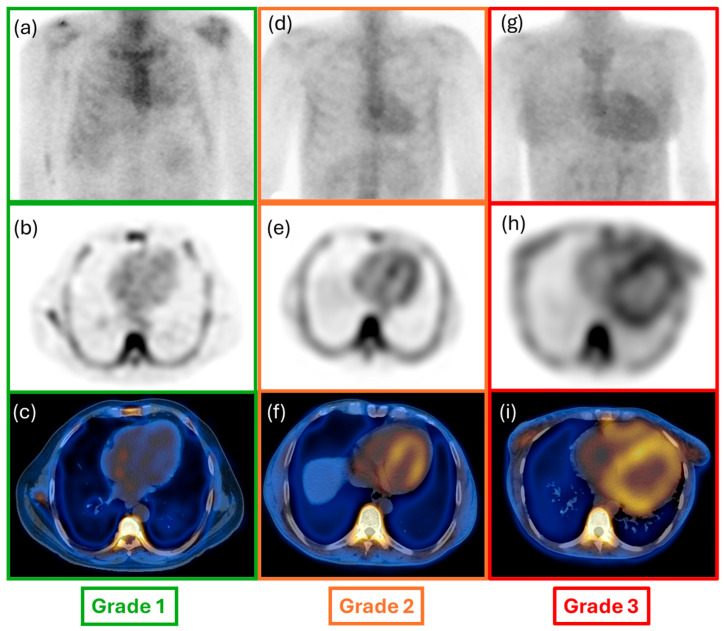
Whole-body and SPECT-CT axial view of a patient presenting cardiac ATTR: (a–c) Perugini Grade 1; (d–f) Perugini Grade 2; and (g–i) Perugini Grade 3.
